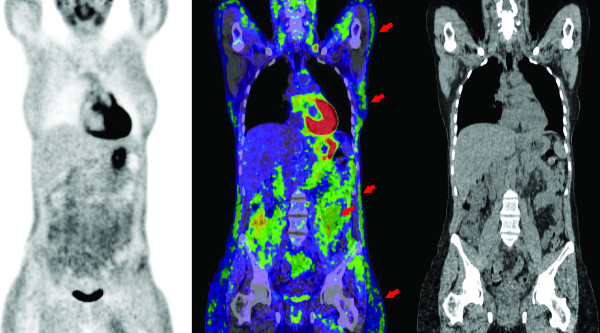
Biodistribution of FDG. Coronal PET, PET/CT fusion, and CT images demonstrating prominent subcutaneous white adipose tissue metabolic activity throughout the thorax, abdomen, and pelvis (arrows). More intense metabolic activity uptake was observed in intra-abdominal fat (arrow). Intense gastric and myocardial uptake combined with low hepatic and negligible muscle uptake was observed.
